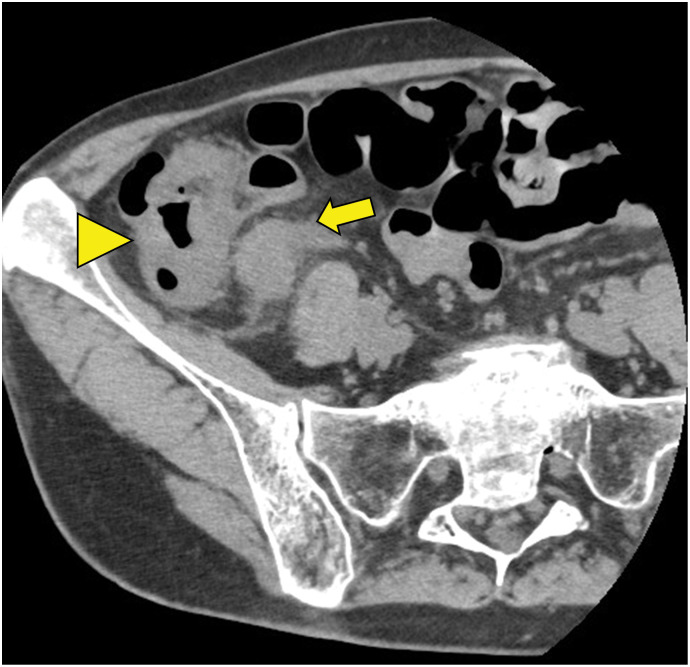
Contrast-enhanced CT. CT shows irregular wall thickening with contrast effect in the ascending colon (arrowhead). Regional lymphadenopathy is evident (arrow).
